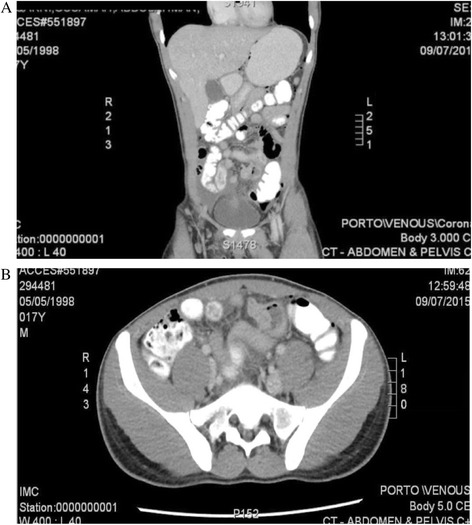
a and b views: CT scan of the abdomen and pelvis, showing an abnormal thickening of an ileal loop with possible presence of a MD. There are multiple air pockets suggesting an area of perforation at the thickened part of the ileal loop. (a: coronal view, and b: sagittal view)
